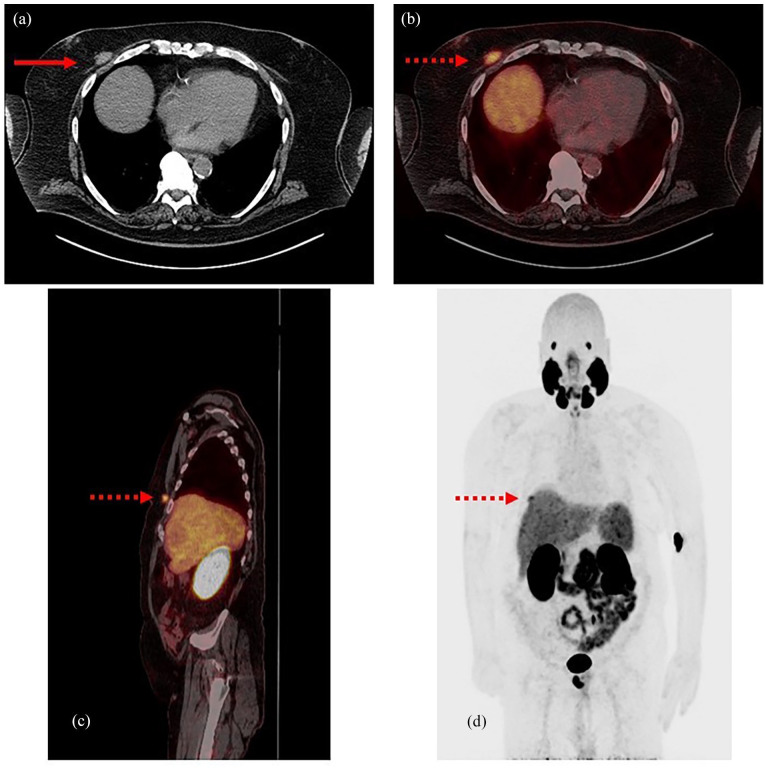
CT and ^18^F-PYLARIFY PSMA-PET images. (a) Noncontrast axial CT image displays a soft tissue mass along the right anterior chest wall abutting the pectoralis muscle (red arrow). Concordant abnormal PSMA radiotracer uptake (SUV_max_ 7.7) is depicted on axial (b) and sagittal fused PET and noncontrast CT (c) images, and on whole body maximum intensity projection image (d) (dashed red arrows). PSMA: prostate-specific membrane antigen; PET: positron emission tomography; CT: computed tomography.
